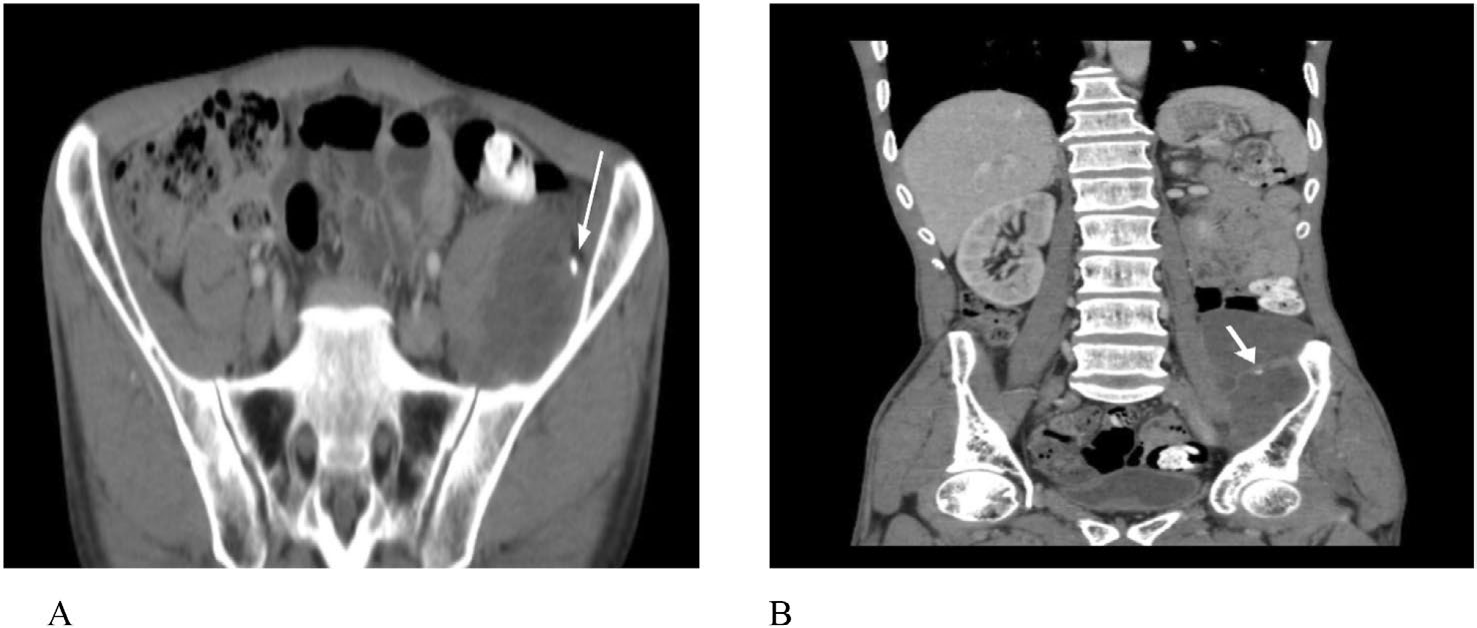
Spiral CT scan of abdomen and pelvis with IV and oral contrast administration. (A) Axial contrast‐enhanced CT image shows thin wall cystic mass in Lt lower quadrant of the abdominal cavity with involvement of Lt iliacus muscle, and foci of calcification in the wall are seen (arrow). (B) Coronal contrast‐enhanced ct image shows a multilocular thin wall cystic mass in the LLQ containing multiple thin septa and foci of calcification in the septa (arrow) with peripheral thin‐rim enhancement in the wall and septa, without a solid component; no free fluid in the abdominopelvic cavity is seen.
